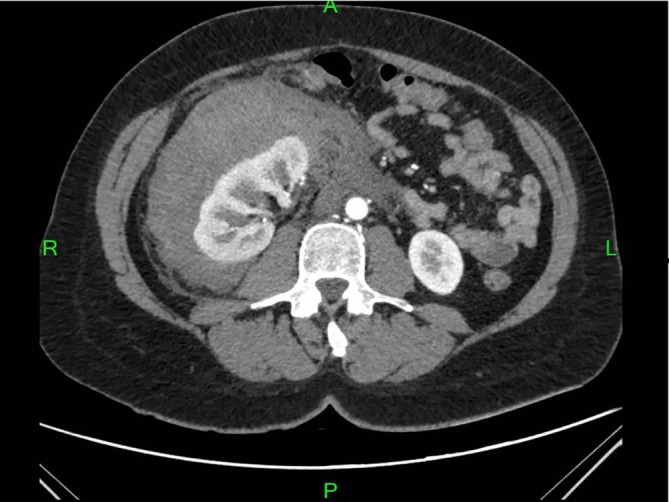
Contrast‐enhanced abdominal CT scan showing a hypodense parenchymal lesion in the right kidney with active contrast extravasation, associated with a large perirenal hematoma extending into the anterior and posterior pararenal spaces, consistent with hemorrhagic renal metastatic involvement.
